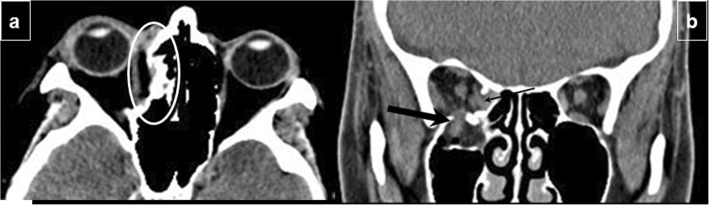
Orbital blowout. a Axial CT image shows disruption of the internal wall of the orbit with fat herniation (circled). b Coronal view shows internal (thin arrow) and inferior (thick arrow) wall disruption; the inferior rectus muscle has herniated and has consequently lost its normal flattened appearance
